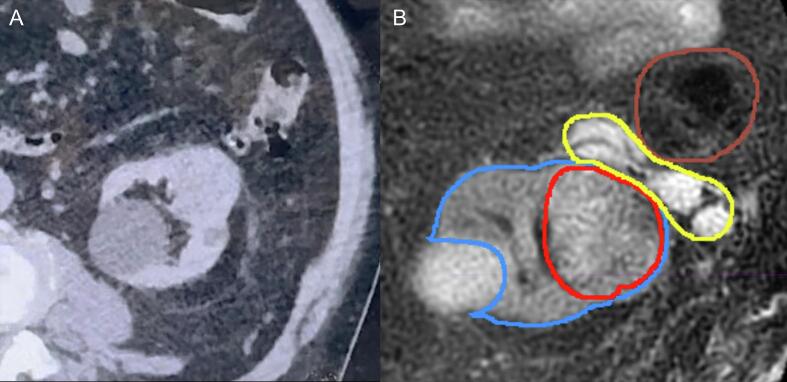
Pre- and post-spacer insertion CT scans for the primary left renal tumor. (A): Pre-spacer insertion CT showing the left renal tumor closely adjacent to the large bowel, with minimal separation. (B): Post-spacer insertion scan with delineation of key structures. The blue outline represents the left kidney. The left renal tumor (outlined in red) is now separated from the large bowel (outlined in brown) by the sHA spacer (outlined in yellow). The spacer creates a safe distance between the large bowel and the high-dose radiation field.
